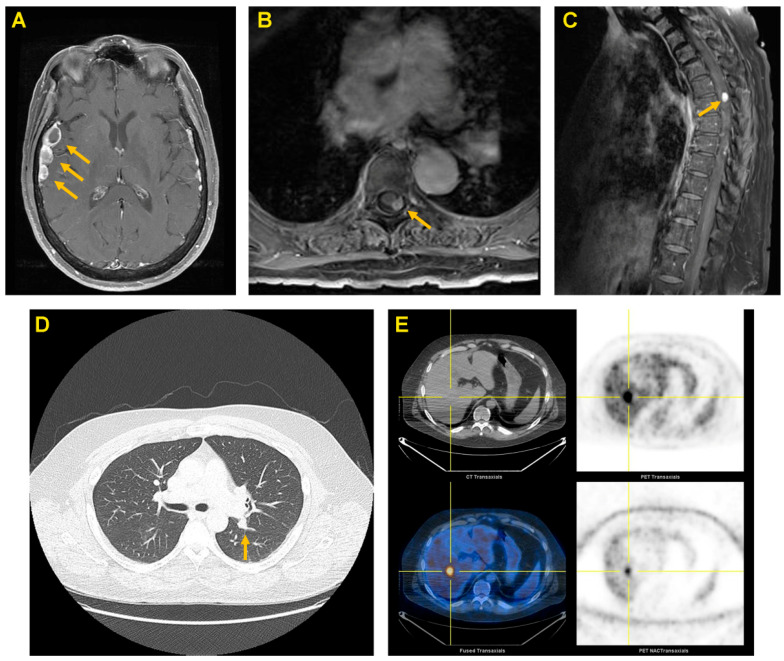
Pathology-proven metastases from pituitary carcinoma to other central nervous system (right temporal dura, spine) and systemic (lungs, liver) locations. (A) T1-weighted post-contrast MRI in the axial plane showing multifocal right temporal dural metastases in patient 12. (B,C) T1-weighted fat-saturated post-contrast MRI in the axial (B) and sagittal (C) plane showing an intradural extramedullary nodule at the T6 level causing asymptomatic cord flattening in patient 19. (D) CT of the chest showing a 1.2 cm nodule (arrow) posterior to the left main pulmonary artery in patient 15. (E) PET/CT showing pituitary carcinoma metastasis in the right lobe of the liver in patient 9.
